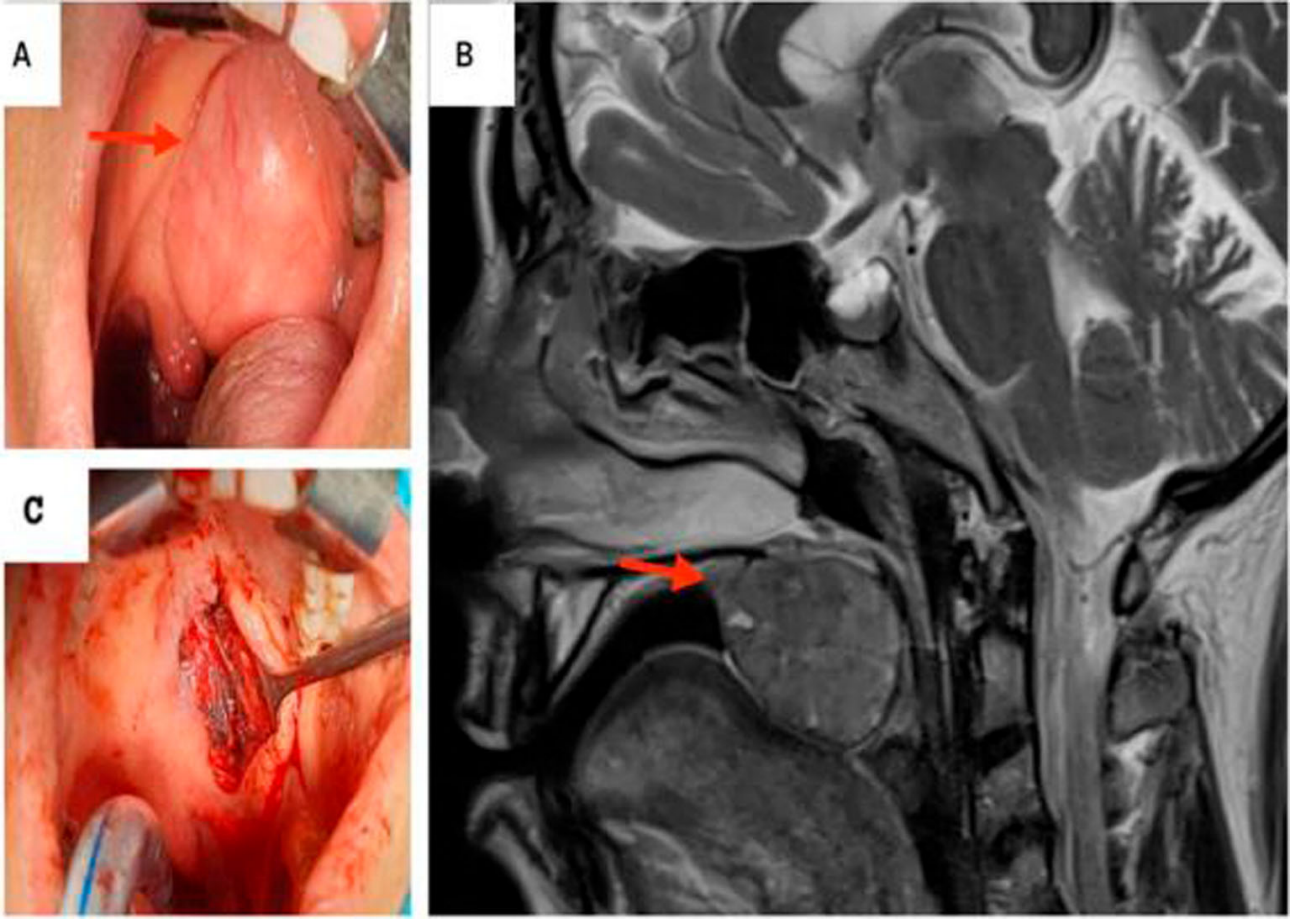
Pleomorphic adenoma of the palate. A: Preoperative view: Exposure of the mass in the palate. B: T2-weighted MRI showing a hyposignal ovoid well-defined mass within the right soft palate. C: Peroperative images: the defect post excision of the mass.
